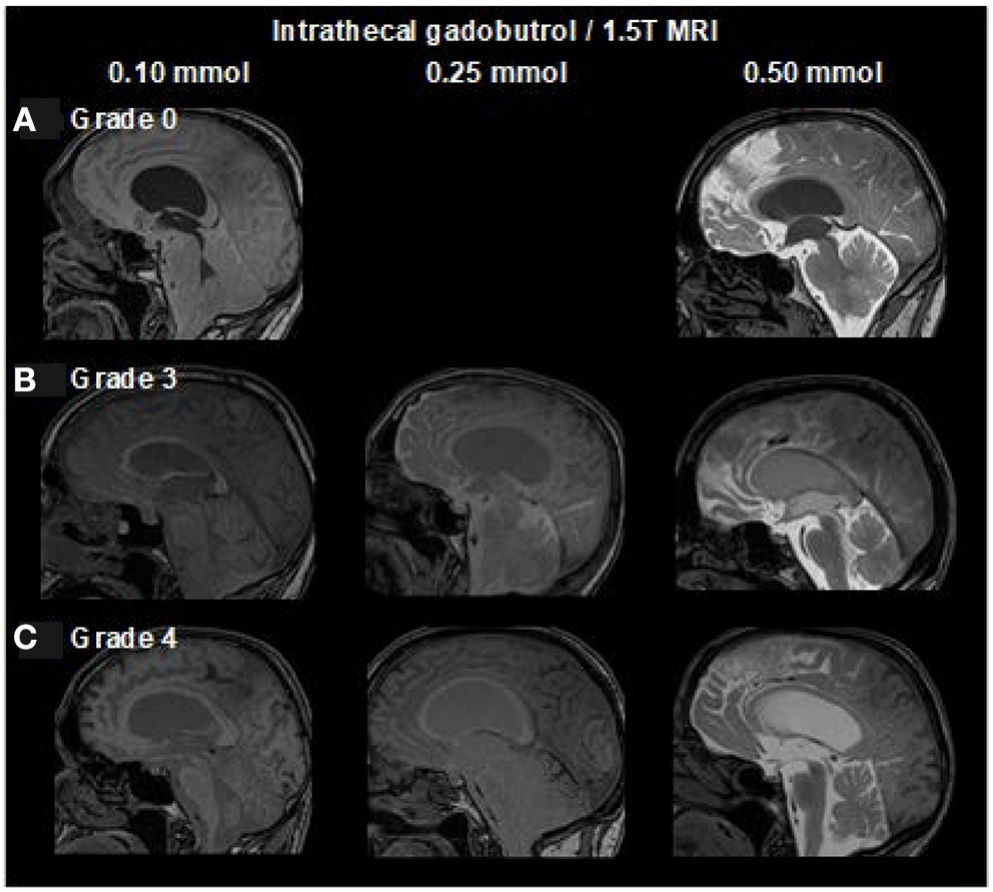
Dose-dependent visualization of ventricular reflux of CSF tracer from sagittal reconstructed T1 weighted images (1.5T MRI) at 24 h after intrathecal gadobutrol in doses of 0.10, 0.25, and 0.50 mmol. Ventricular reflux is categorized into five categories: (A) Grade 0: No supra-aqueductal reflux. Grade 1: Sign of supra-aqueductal reflux Day 1. Grade 2: Transient enrichment of lateral ventricles Day 1. (B) Grade 3: Lasting enrichment of lateral ventricles Day 2 (not isointense with CSF subarachnoid). (C) Grade 4: Lasting enrichment of lateral ventricles Day 2 (isointense with CSF subarachnoid). At 1.5T, MRI was not obtained post-contrast at Day 1, which allowed for scoring of grades 0, 3, and 4 only. In iNPH, we only consider grades 3 to 4 Day 2 at 24 h as abnormal (18).
